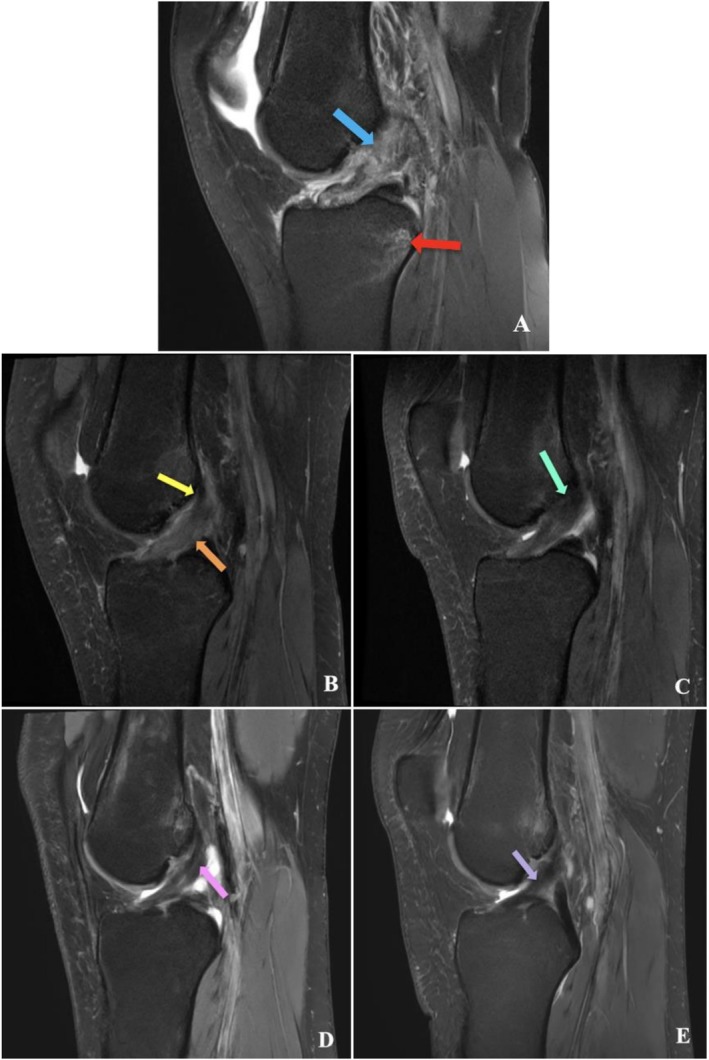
Proton density fat saturated (PD‐FS) sequence MRI of the left knee in the sagittal plane of a 20‐year‐old female athlete with complete ACL rupture at day 3 post injury. (A) showing ACL fiber discontinuity (blue arrow) and pivot shift pattern of bone marrow oedema signal (red arrow). Follow up imaging was completed at 3 months (B), showing reduced thickness at the femoral ACL attachment (yellow arrow) with a sag in the ACL (orange arrow); at 6 months (C), showing minimal reduction in the thickness of the ACL at the femoral attachment (teal arrow); at 12 months (D), showing a taut ACL with low signal intensity along the length of the ACL (pink arrow); and at 36 months (E), showing an intact, taut ACL with normal low signal intensity (purple arrow).
